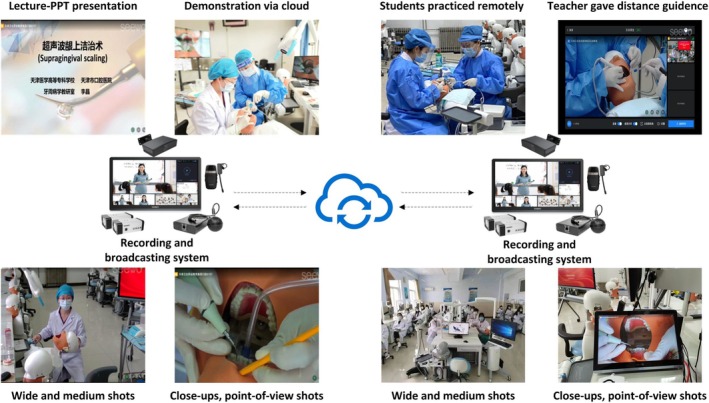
The hybrid sim‐lab ultrasonic supragingival scaling course. The teacher delivered lectures and demonstrations with the assistance of the recording and broadcasting system. The presentation included wide shots, medium shots, close‐ups and point‐of‐view shots as appropriate. Following this, students engaged in remote practice under the same recording and broadcasting system. The teacher provided guidance to students from a distance, and mutual interaction occurred without any barriers.
